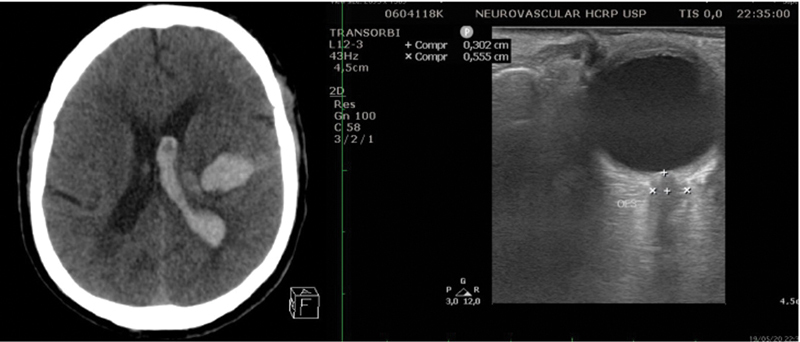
Baseline bedside ONUS on patient admission. (A) Cranial NCCT scan depicting a left ICH with volume of 11 ml and extensive left lateral ventricular hemorrhage. (B) ONUS showing ipsilateral dilated ONSD. Abbreviations: ICH, intracerebral hemorrhage; NCCT, non-contrast computed tomography; ONSD, optic nerve sheath diameter; ONUS, optic nerve ultrasonography.
